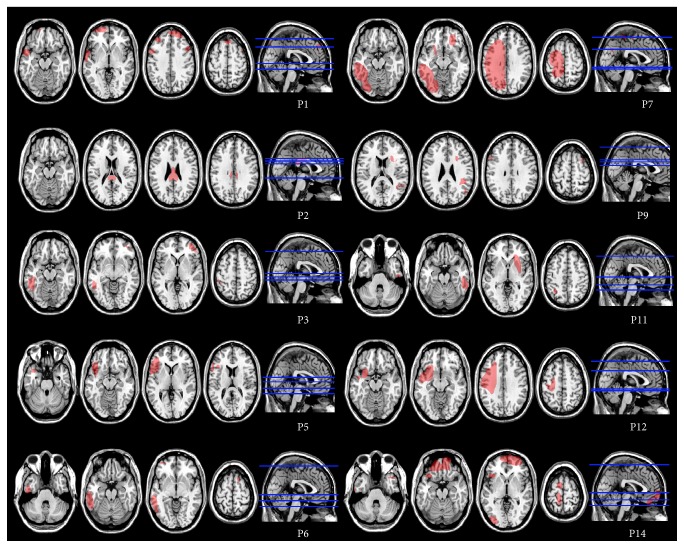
Lesion reconstruction images from MRI or CT scans projected onto the normalized MNI template for the 10 TBI patients with detectable focal lesions.
